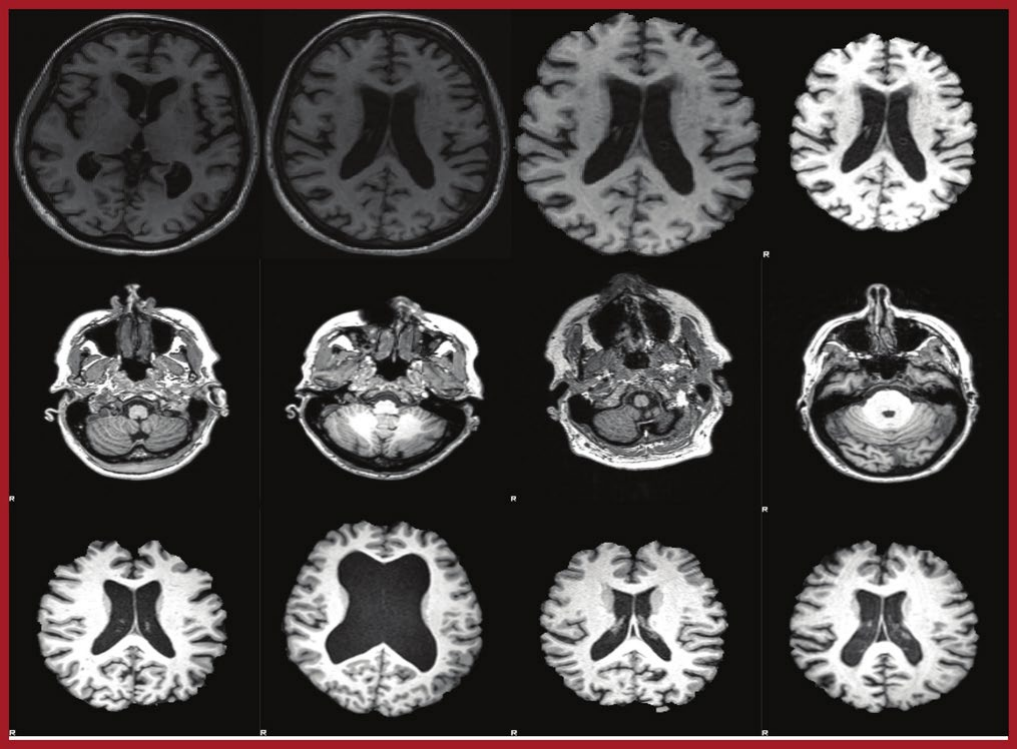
First Row – Intermediate Stages of Preprocessing: (i) reorientation, (ii) registration, (iii) skull-stripping, (iv) histogram equalisation. Second Row – Sample raw MRI scans from the ADNI cohorts. Third Row – Corresponding final preprocessed output
